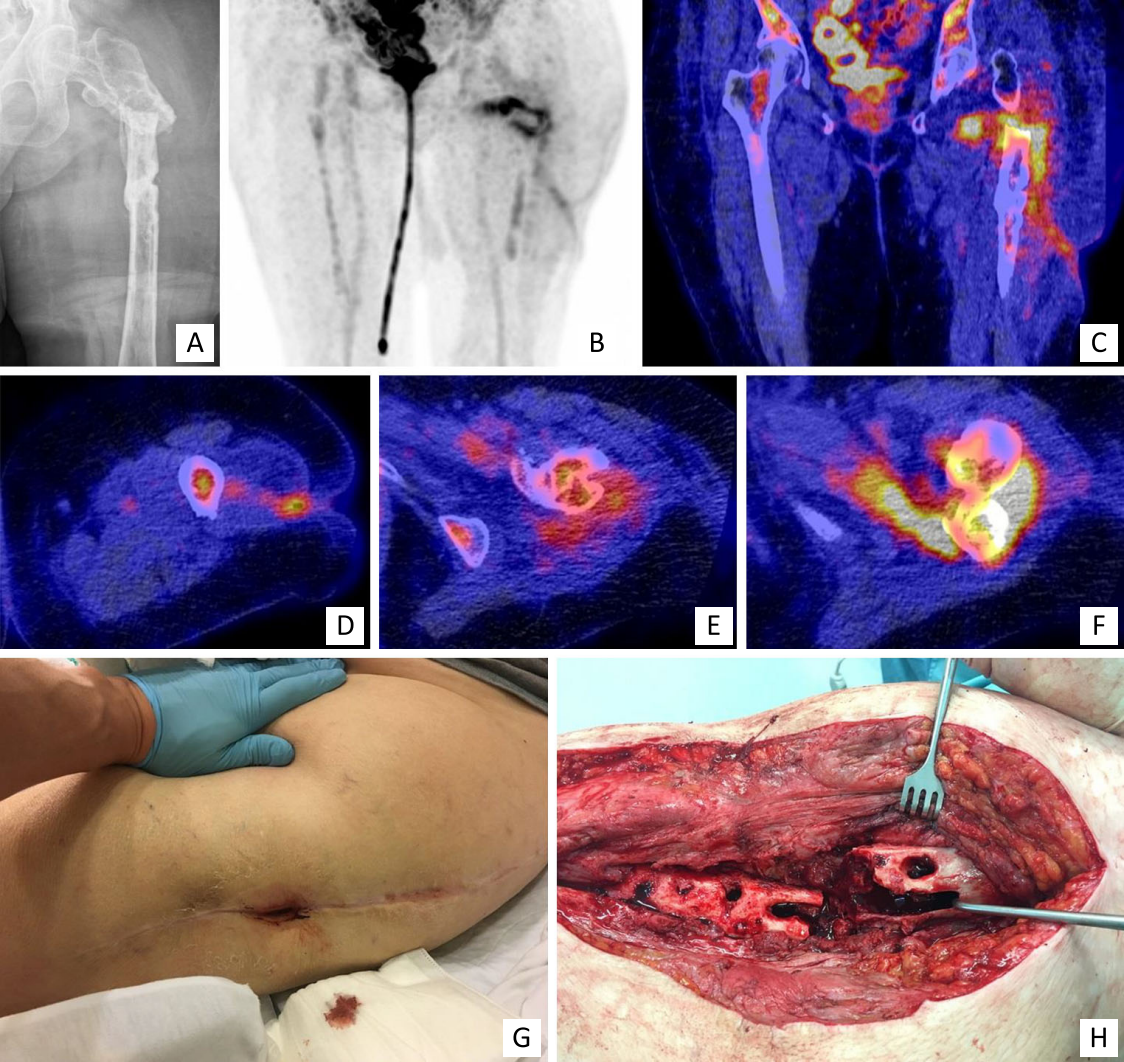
Clinical example of FDG-PET/CT. A 77-year-old woman who had a proximal femur fracture for which she underwent open reduction and internal fixation with a femur plate which had to be removed at a later stage due to infection. a X-ray, AP view: no consolidation, severe angulation, heterogeneous sclerotic aspect around the fracture. She was referred to our hospital with a fistula in the lateral thigh and a clinical suspicion of osteomyelitis of the proximal femur. Further imaging demonstrated an infection of the proximal femur, a medial abscess and a fistula coursing to the lateral aspect of the thigh which correlated with the clinical findings during surgery. b–f
